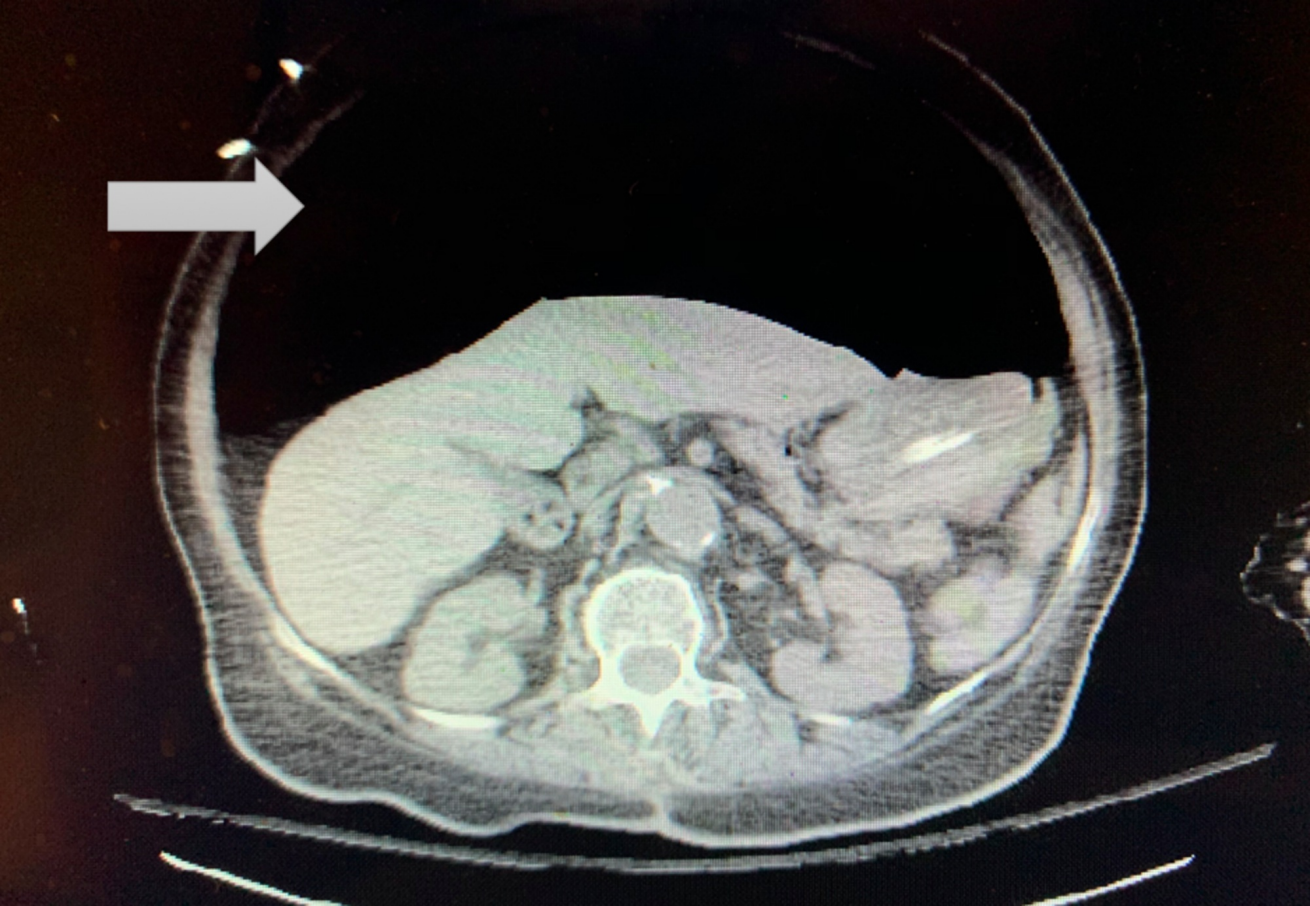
CT scan of abdomen shows massive pneumoperitoneum
